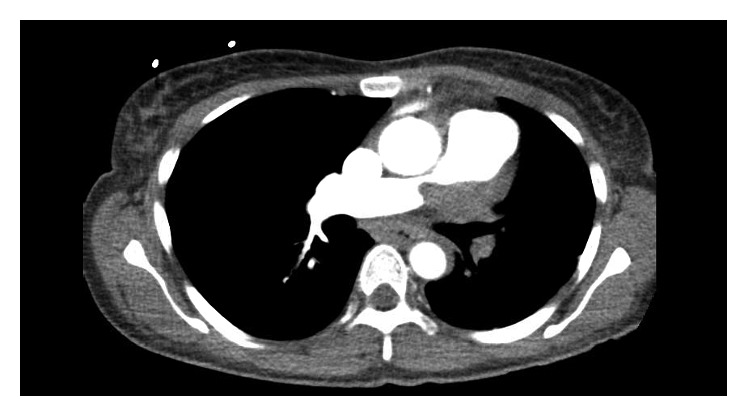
CT scan showing mediastinal mass narrowing the right pulmonary artery with full occlusion of the left pulmonary artery.
